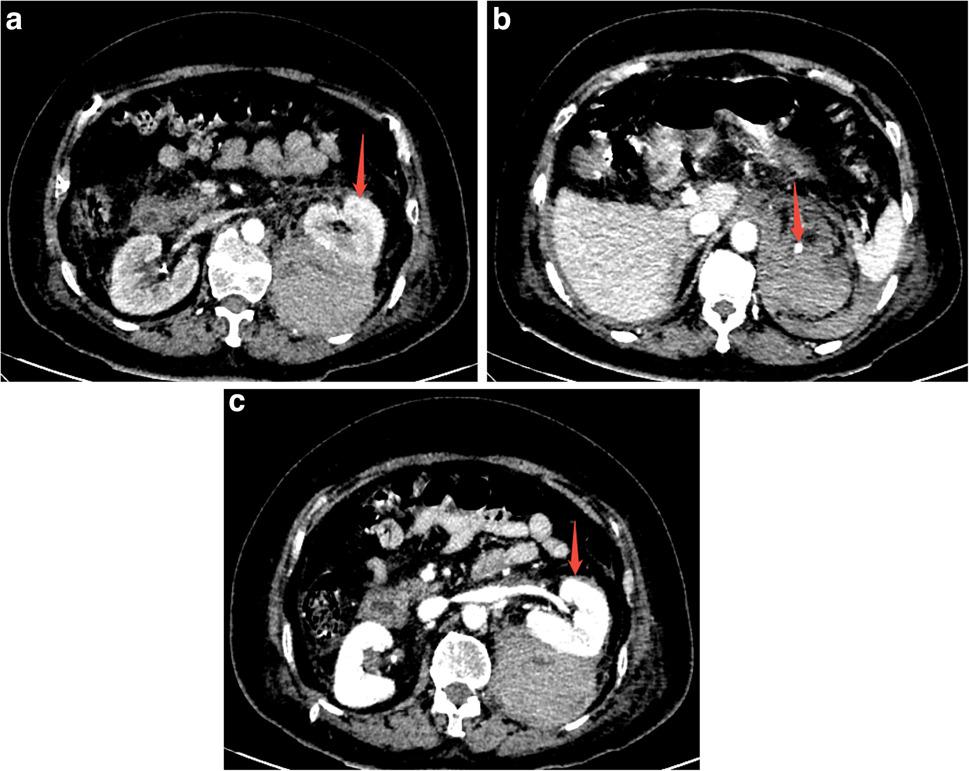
a Contrast-enhanced abdominal CT scan after hemorrhage. Arterial-phase enhancement is observed in the left kidney, indicated by the red arrow, suggesting an active renal parenchymal lesion following the bleeding episode. b Venous-phase contrast-enhanced CT scan after hemorrhage. The venous-phase image shows a focal area of persistent enhancement in the left kidney (red arrow), corresponding to the suspected bleeding site. c Venous-phase contrast-enhanced CT scan after hemorrhage. A venous-phase image demonstrates a persistently enhanced focus in the left kidney (red arrow), consistent with an active or recent bleeding site
